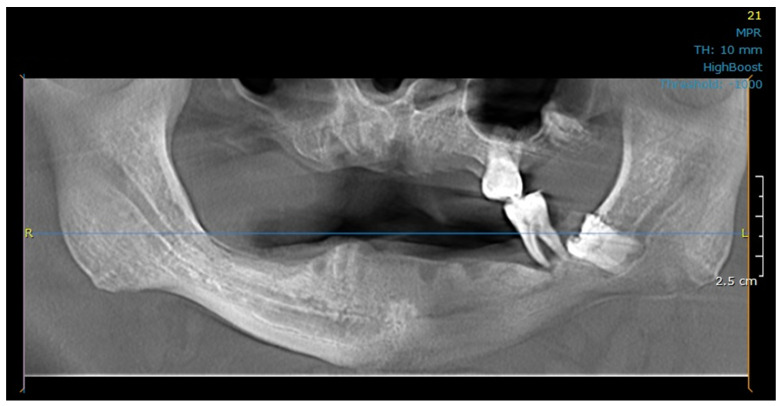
Cone-beam CT (CBCT) image corresponding to the patient’s baseline state.
